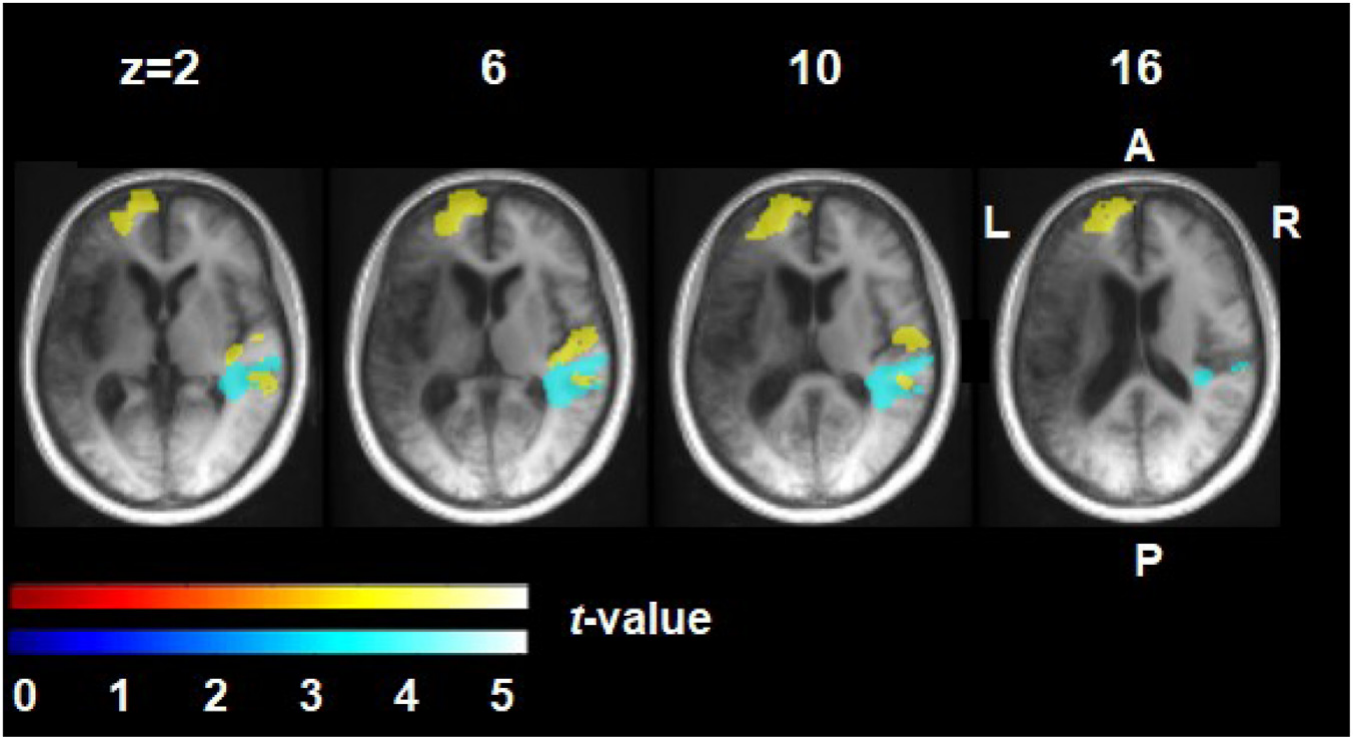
Significant brain clusters with increased volume post-therapy. The brain template is the average T1-weighted MRI image for the 17 participants at T2. A = anterior; P = posterior; L = left; R = right. Yellow = grey matter and blue = white matter. Numbers are MNI coordinates.
